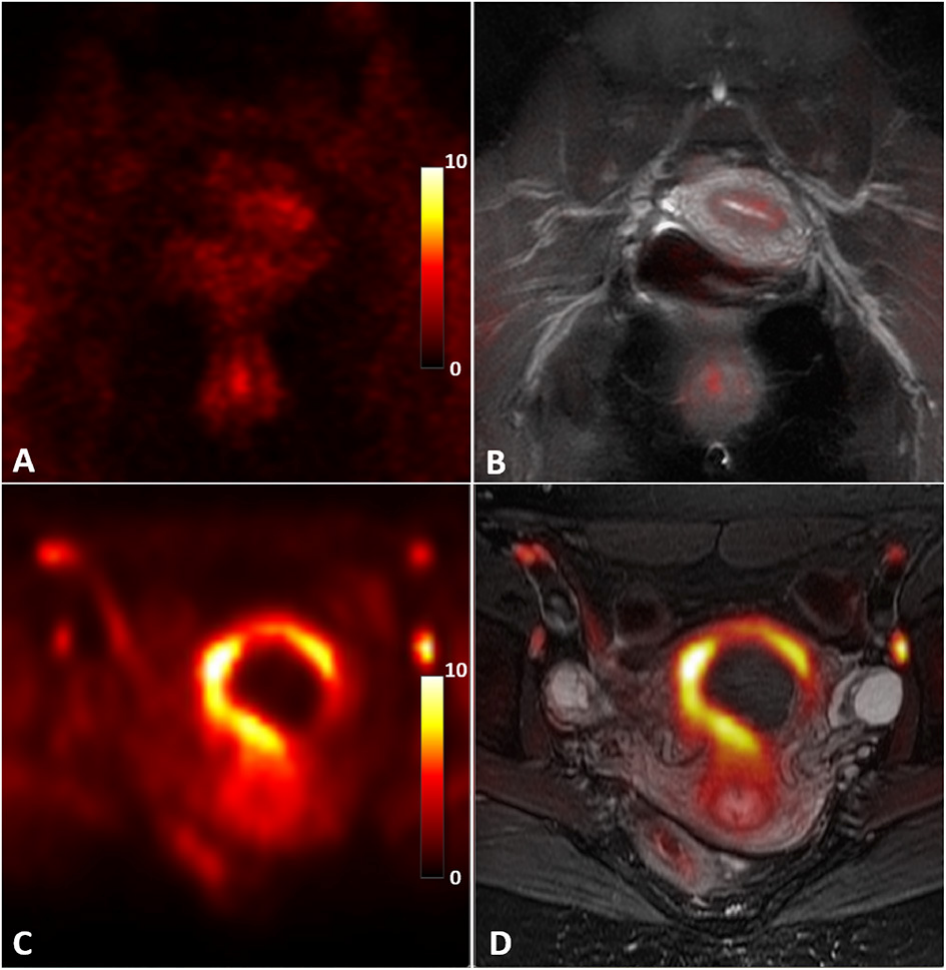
Abnormal S1R tracer uptake of the uterus in Patient 1 compared to an asymptomatic control. S1R PET (A) and PET/MR co-registered (B) images in an asymptomatic control present diffuse, low uptake compared to the S1R PET (C) and PET/MR co-registered (D) images of the patient. Note that the fibroid does not take up the radioligand. The PET images were plotted in the unit of standardized uptake value (SUV).
